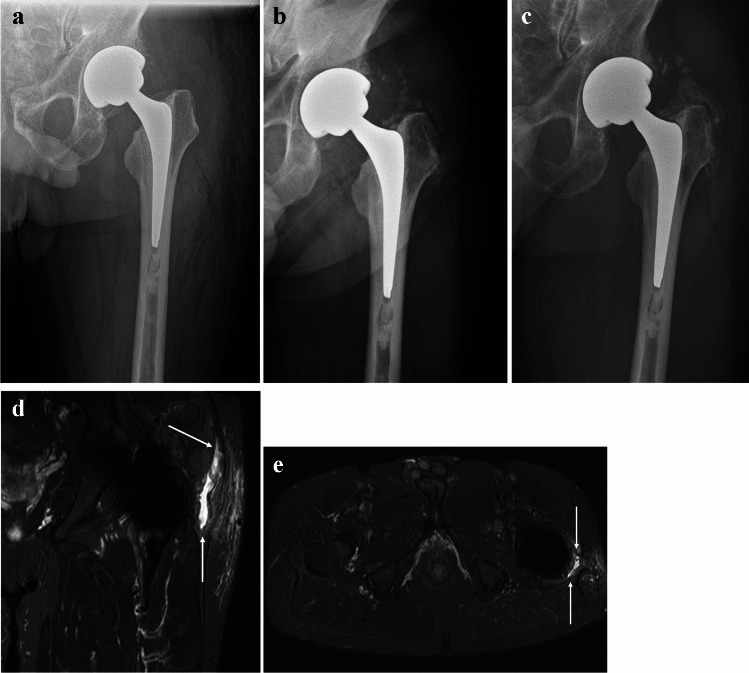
Heterotopic ossification development in a patient. AP radiograph a immediately postoperatively, b 3 months after THA presenting Brooker grade I, and c 12 months after THA presenting Brooker grade III. MRI of the same patient 3 months after THA, showing the active phase of calcified tendinitis in d the STIR coronal and e the STIR axial plane
